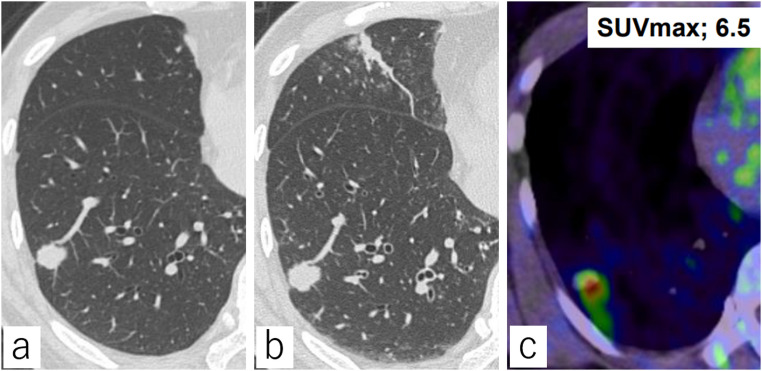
Representative case with radiological features suggesting high tumor aggressiveness. (a) A 48-year-old man with a 1.7-cm pure solid tumor located in the right S9 at initial presentation. (b) The tumor demonstrated rapid growth, increasing to 2.0 cm within one month. (c) Fluorodeoxyglucose (FDG) positron emission tomography showed high uptake (SUVmax, 6.5). He underwent a right lower lobectomy and has remained free from recurrence for 124 months
